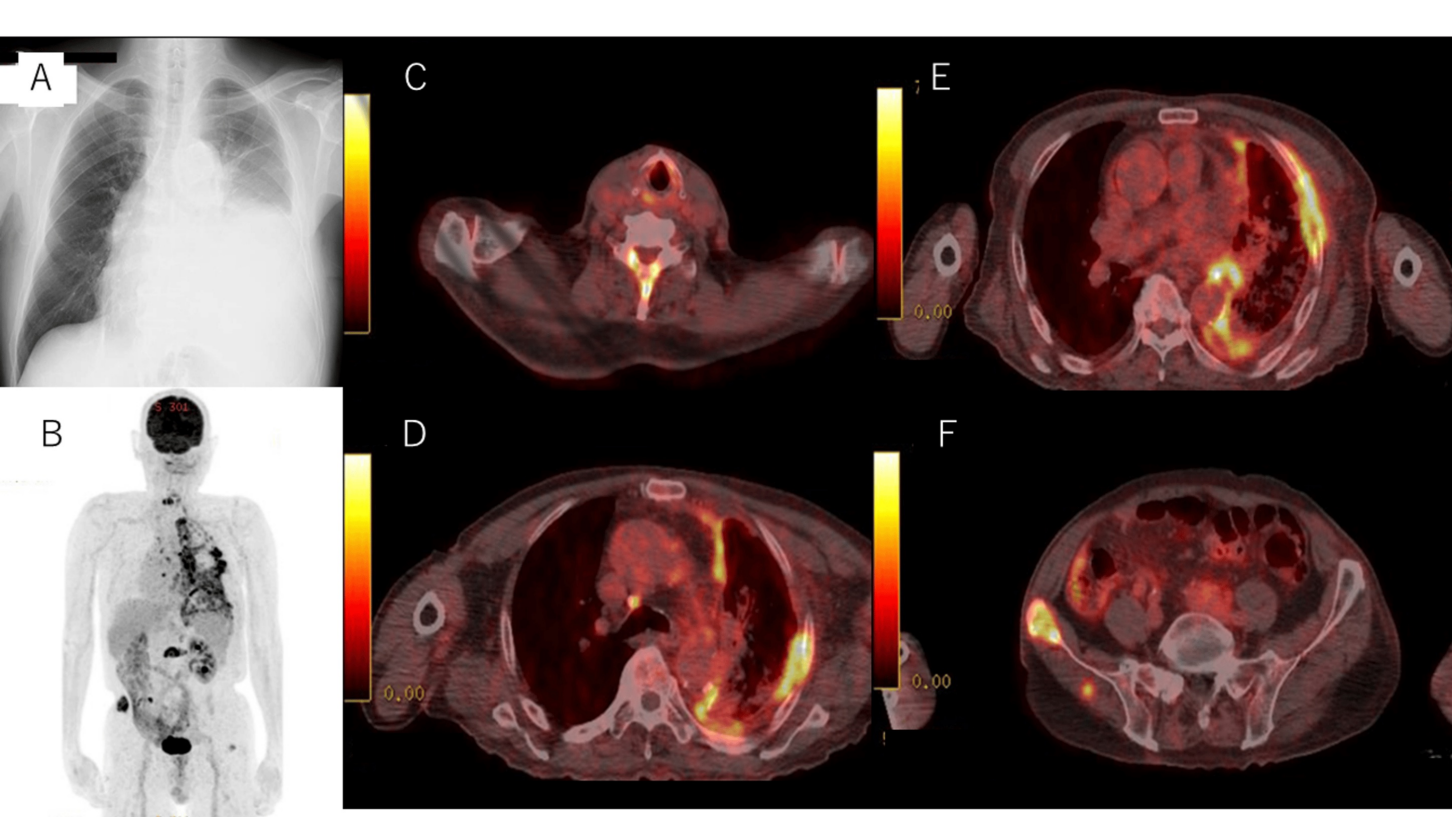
Imaging at recurrence A:  Left pleural effusion on chest radiography. B-F: Fluorodeoxyglucose positron emission tomography shows pleural dissemination and multiple bone metastases.
